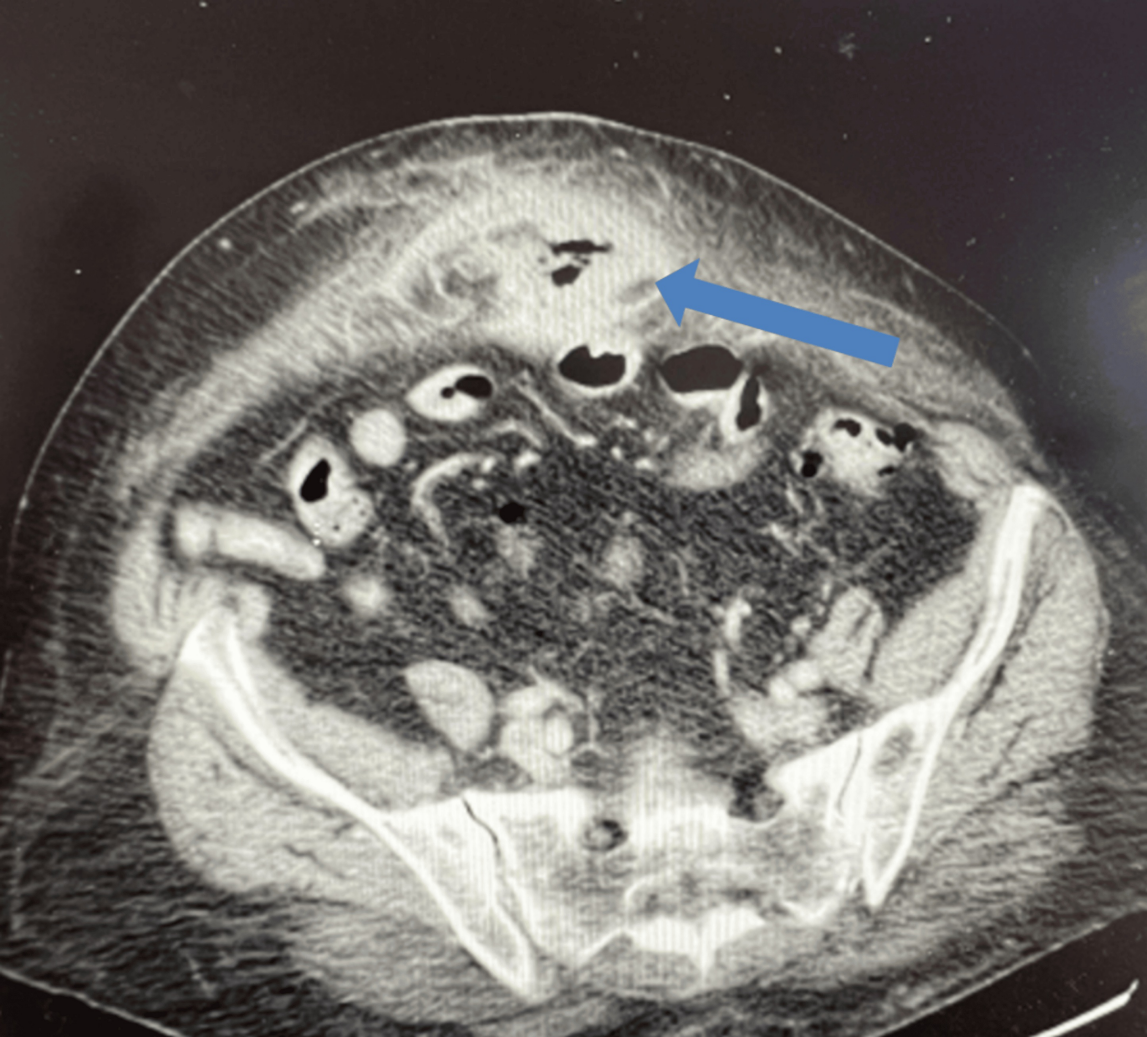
Preoperative CT scan, axial view Blue arrow pointing to peripherally enhancing air and fluid containing collection within the hernia measuring up to 5 x 3 x 3 cm
